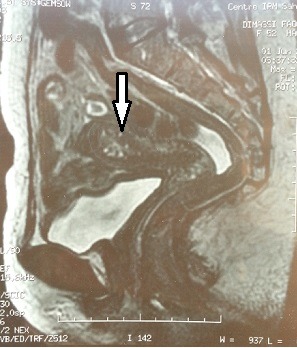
Pelvic magnetic resonance imaging revealed tumor infiltration of the endometrium less than 50% of the myometrium
